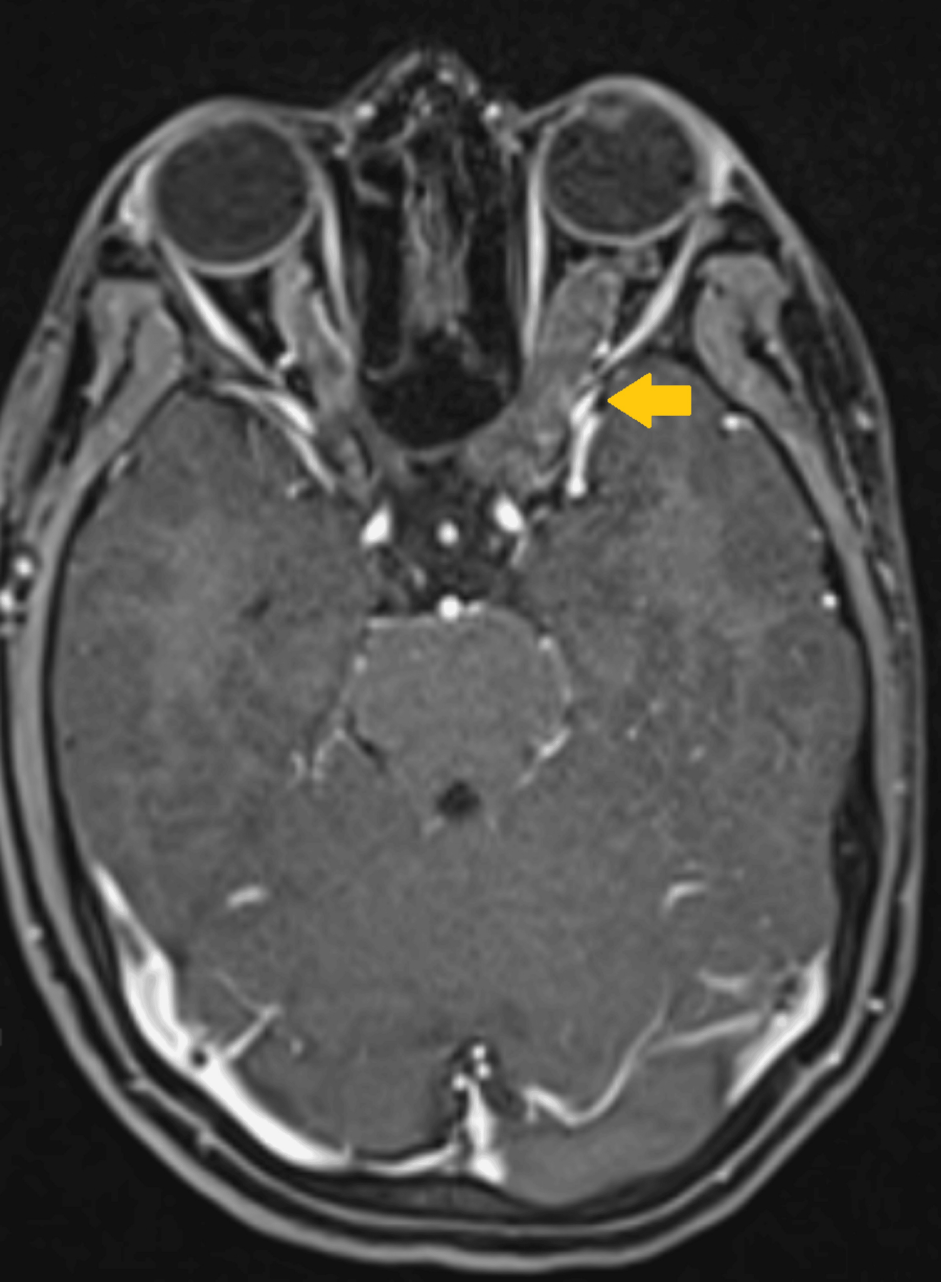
Axial section of the contrast-enhanced MRI of the brain showing fusiform enlargement of the left optic nerve (highlighted with arrow).
